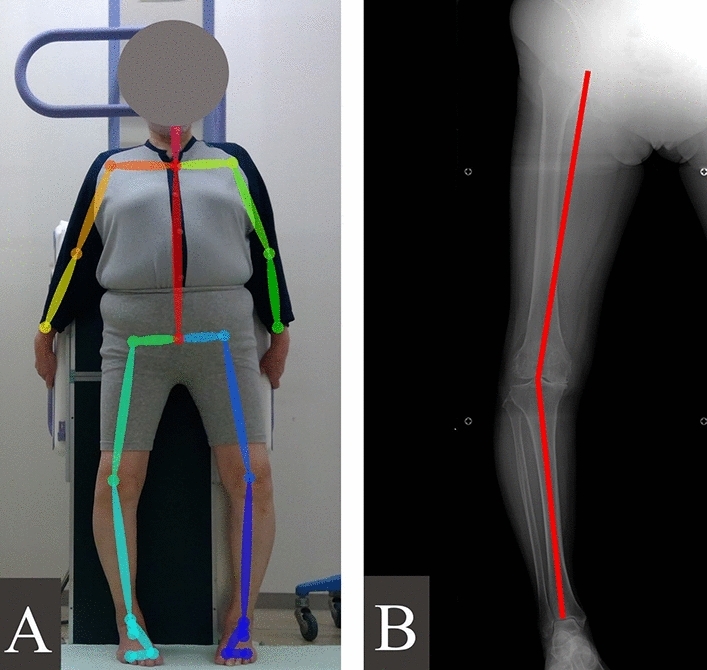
Images for hip-knee-ankle angle measurement. (A) OpenPose image. (B) Radiographic image.
